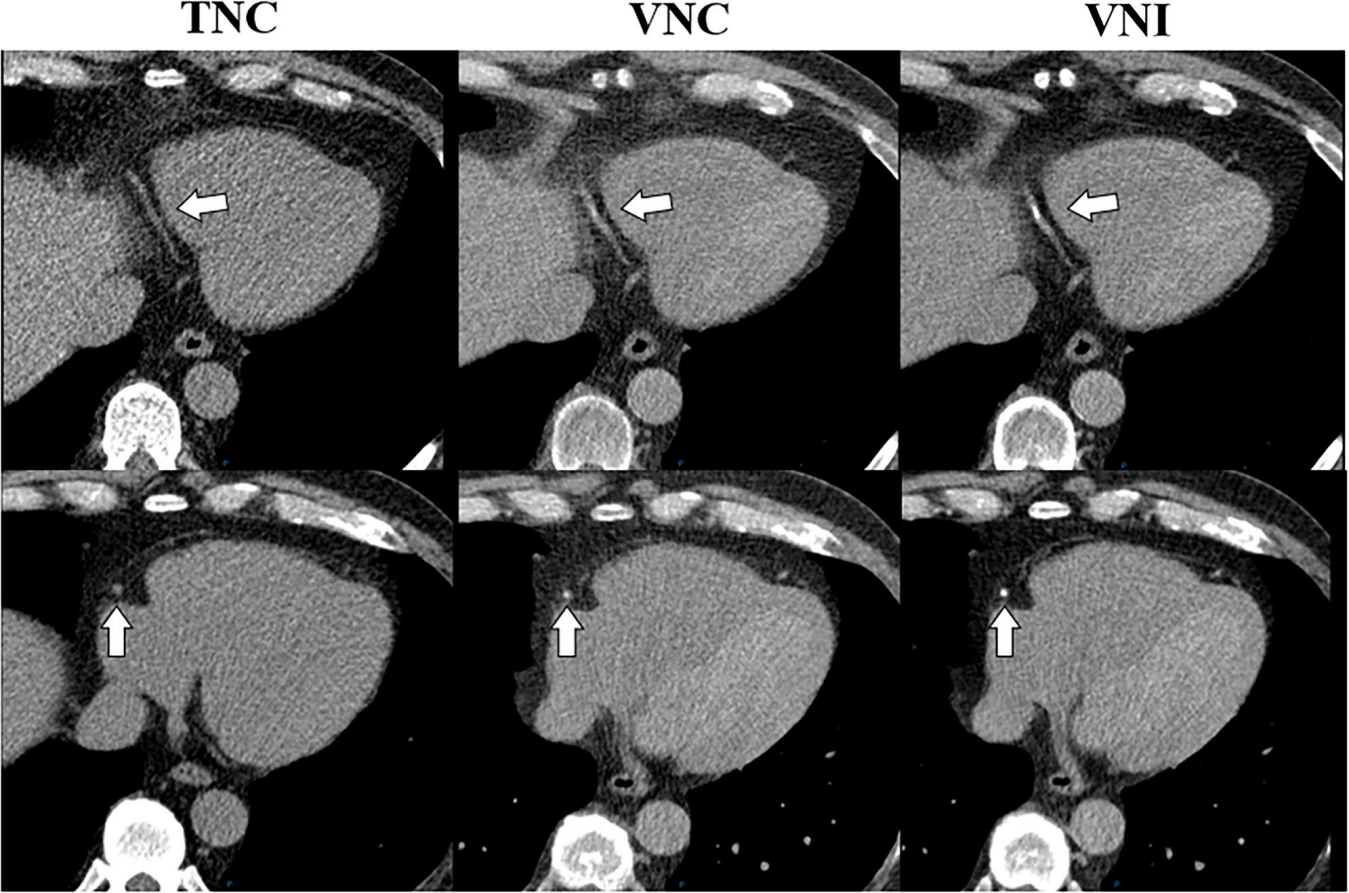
Calcium scoring on TNC, VNC, and VNI in the right coronary artery. A 63-year-old male patient suspected of coronary artery disease underwent calcium scoring and CTA. No calcifications in the RCA were present on the TNC images, while calcifications were detected on both VNC and VNI
